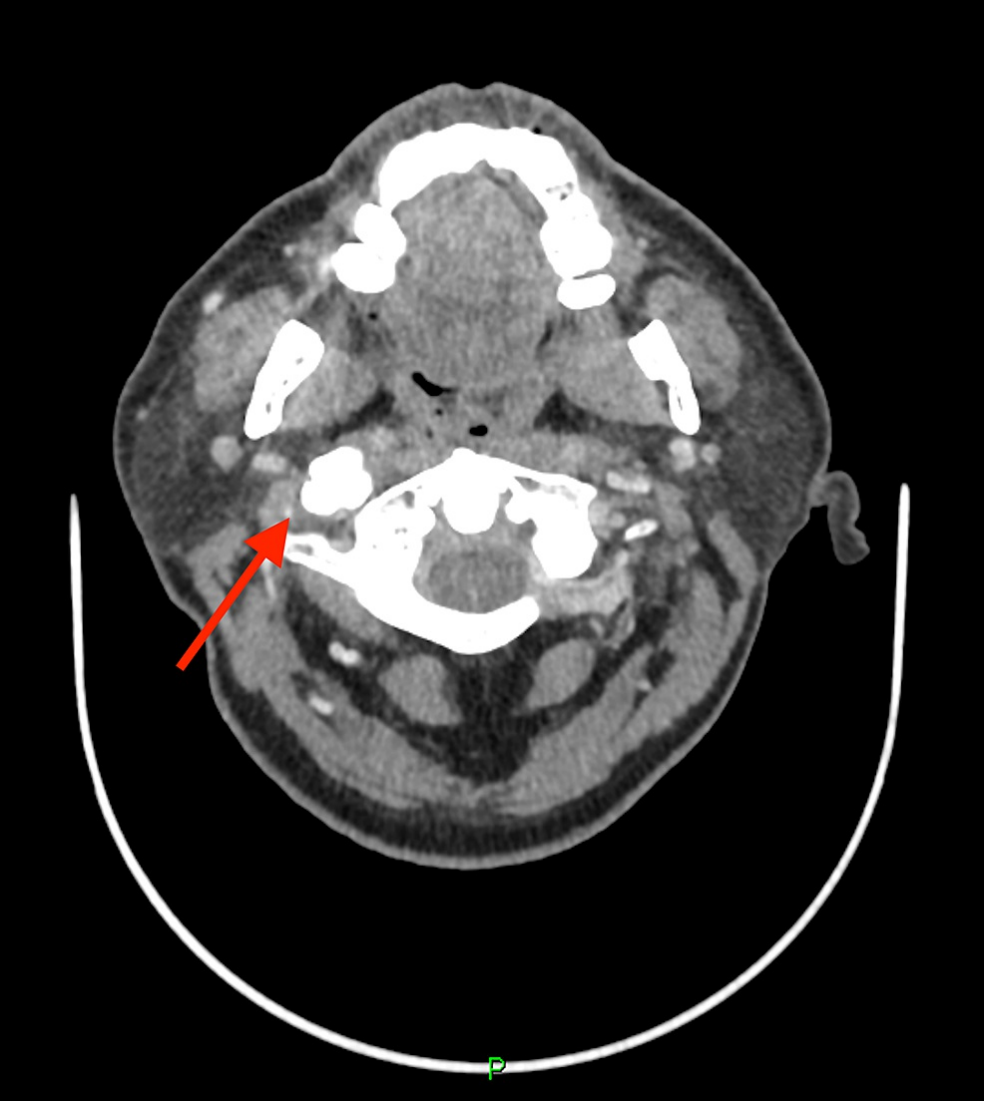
Follow-up CT of the neck with contrast (axial plane) showing calcification of the elongated right stylohyoid ligament (red arrow).
